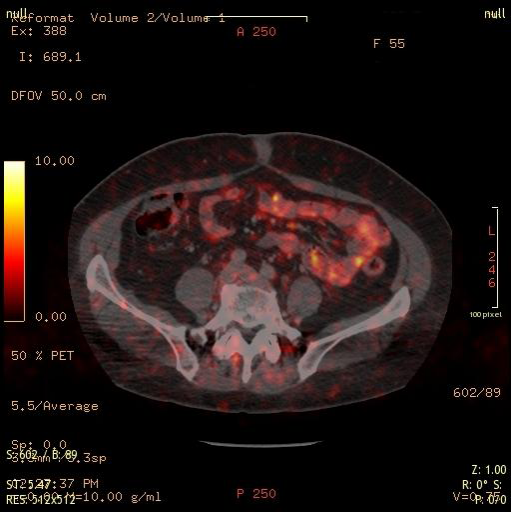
PET/CT Ga^68^-TOC uptake in intestinal structures, in abdominal muscles, and abdominal fatty tissue. The image was taken from a 56-year-old female patient with a NET.
